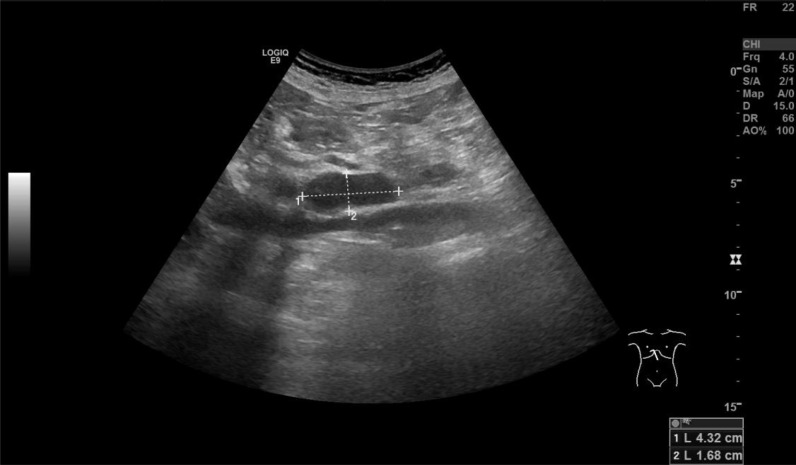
Retroperitoneal lymph node ultrasonography for the first time, the largest mase was measured at approximately 4.0×2.0 cm.
